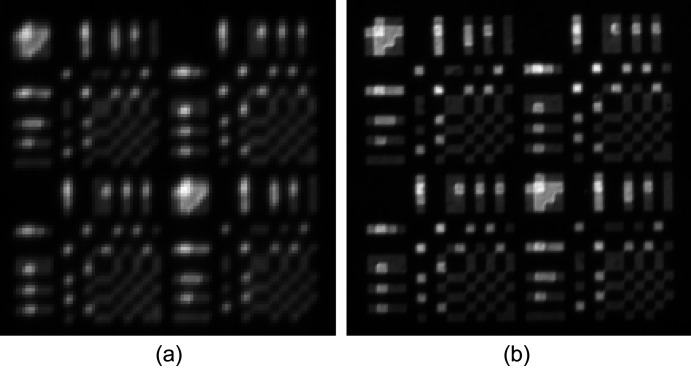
(*a*) Image of X-ray transmission through the [*P*, *N*/*N*, *P*] fabricated 15 × 15 gray-level masks with (*a*) 10 µm pixels and (*b*) 20 µm pixels.
